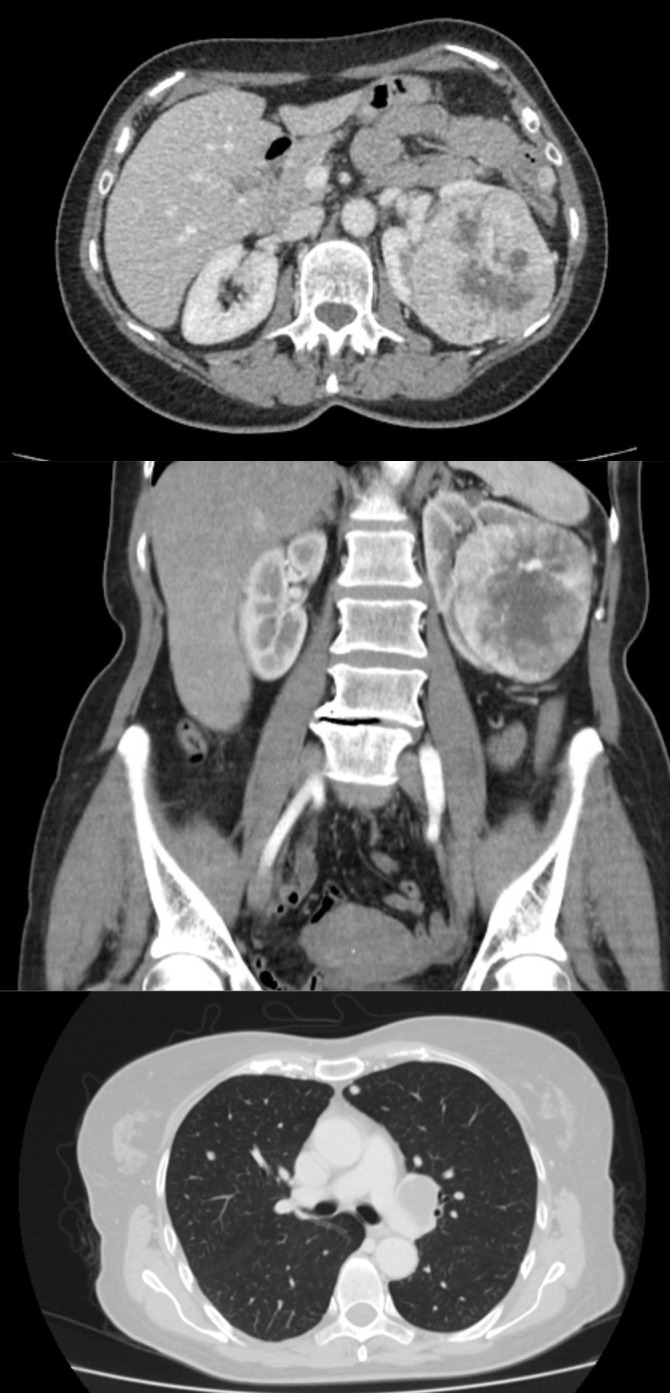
Baseline CT images showing the left renal primary tumor, a largely necrotic 9 × 7 cm mass, in both axial (top) and coronal (middle) planes, along with a peritoneal metastasis in the left paracolic gutter and bilateral pulmonary metastases (axial, bottom).
